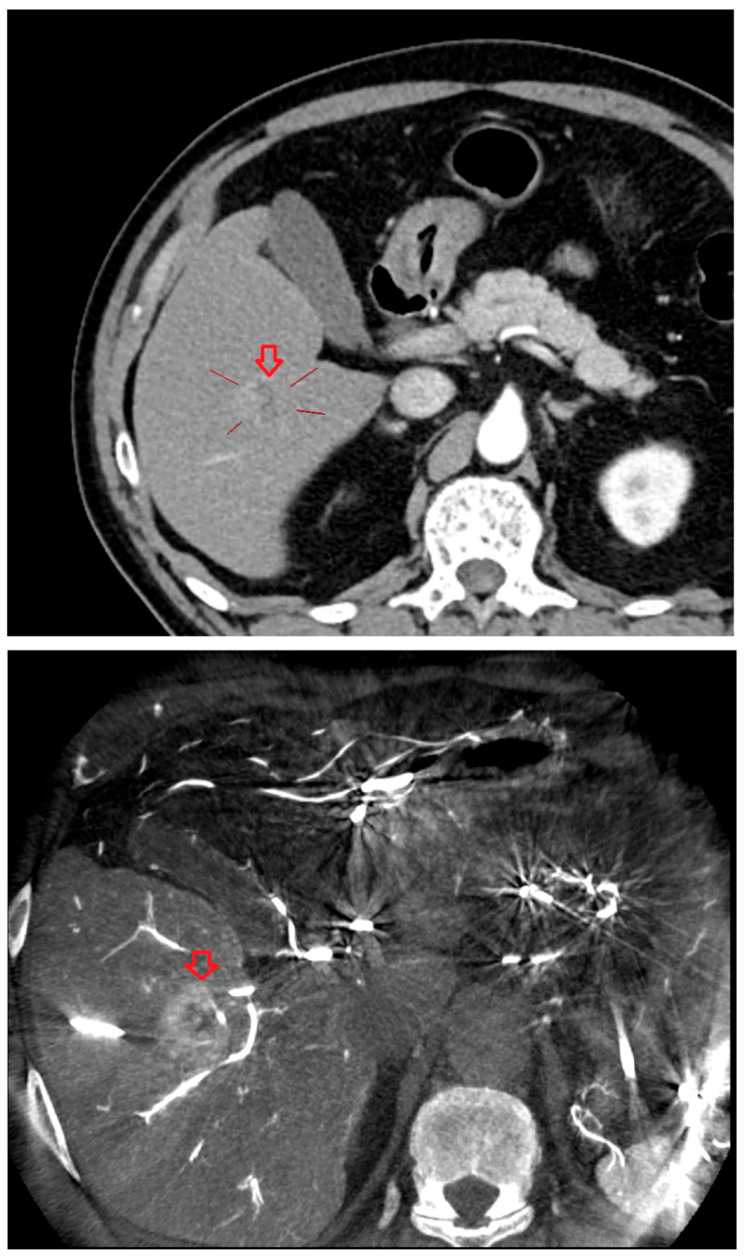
CT images from other patient with hepatocellular carcinoma undergoing angio CBCT-guided RFA. (Top): pre-procedural CT (arterial phase). Red arrow and line = HCC mass (lesion 74.29 HU). (Bottom): intraprocedural angio CBCT. Red arrow = HCC mass (lesion 368.49 HU). ΔHU = 173.9 HU. (intra lesion − intra background) − (pre lesion − pre background).
